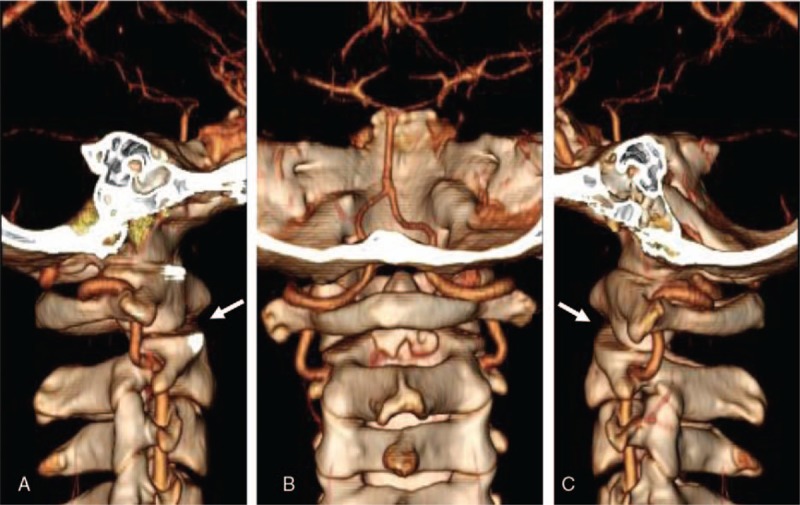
Neck angio-CT scans showing a normal vertebral artery and reduction of C1–2 posterolateral dislocation with type II dens fracture.
